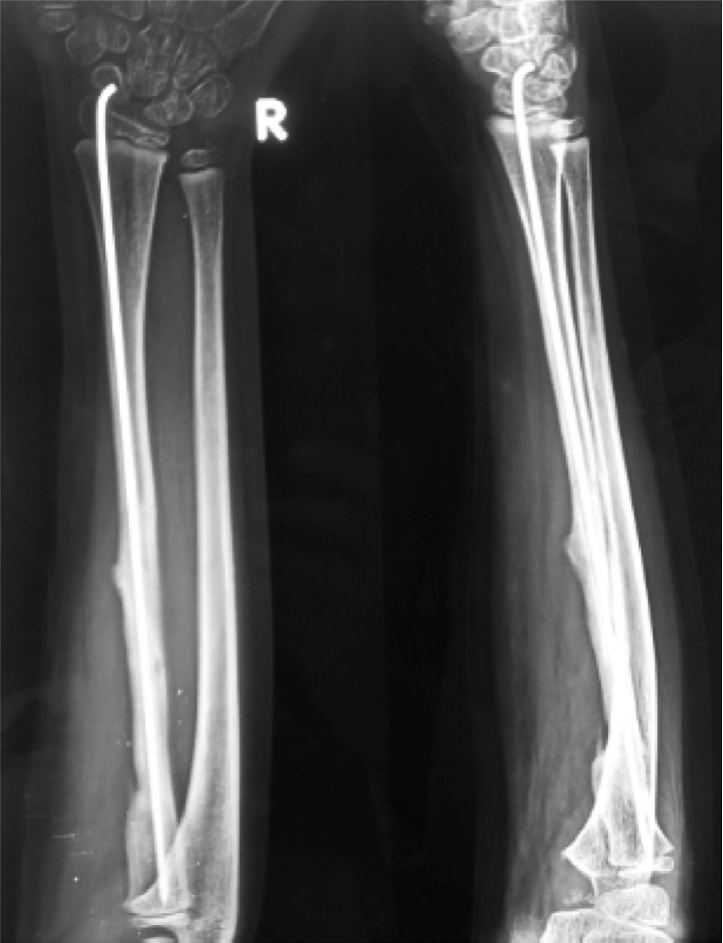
Six months follow-up X-ray showing incorporated graft with K-wire in situ after which the K-wire was removed.
